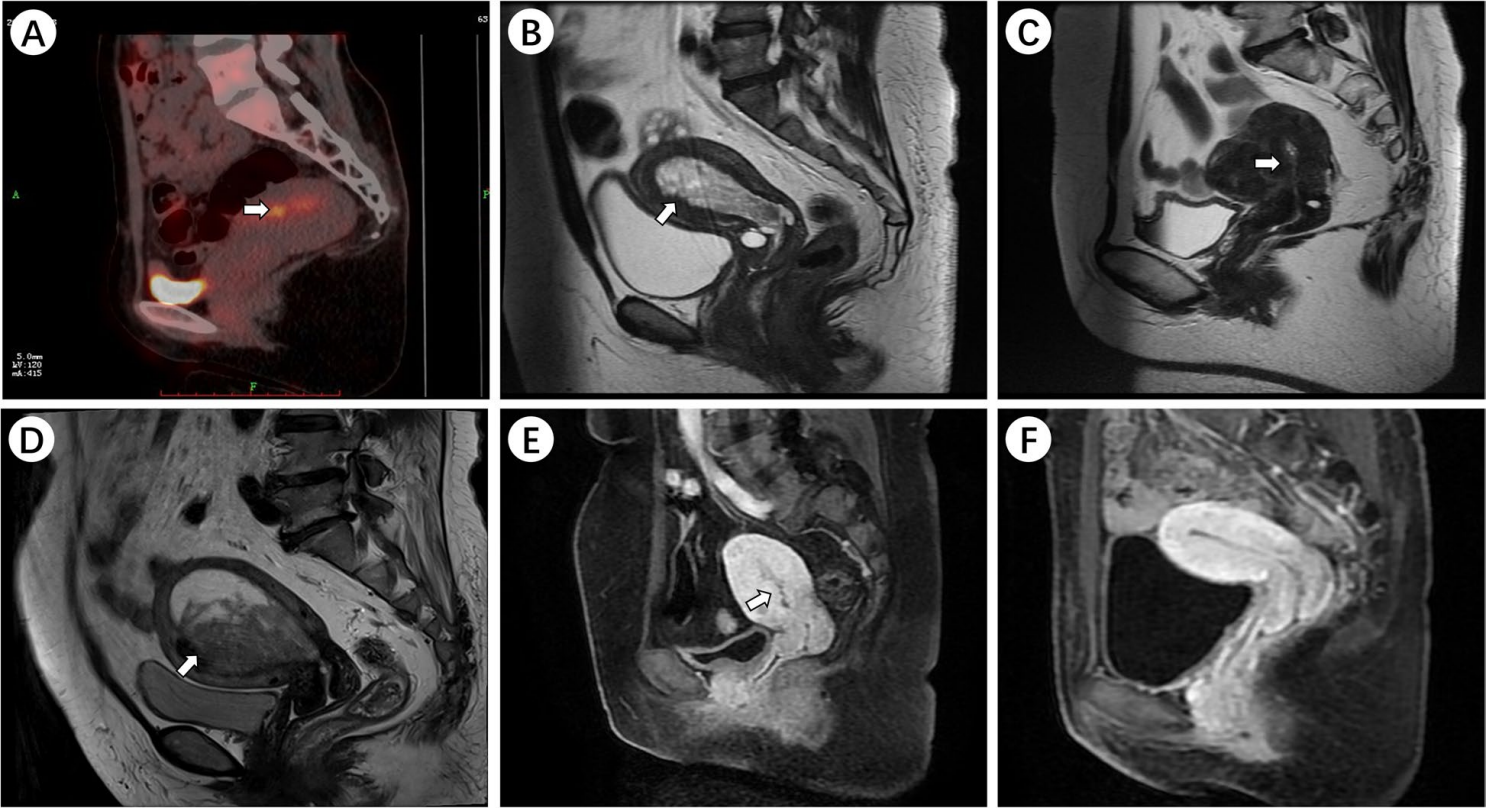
UTROSCT (arrow) in different images of PET-CT and MRI. A A max SUV was 4.5 in the myometrium. B–D UTROSCT is mildly hypointense compared to endometrium signals on sagittal T2 sequence. E UTROSCT is similar to uterus signal and well-delineated on dynamic contrast-enhanced sequence. F No mass observed after curettage
